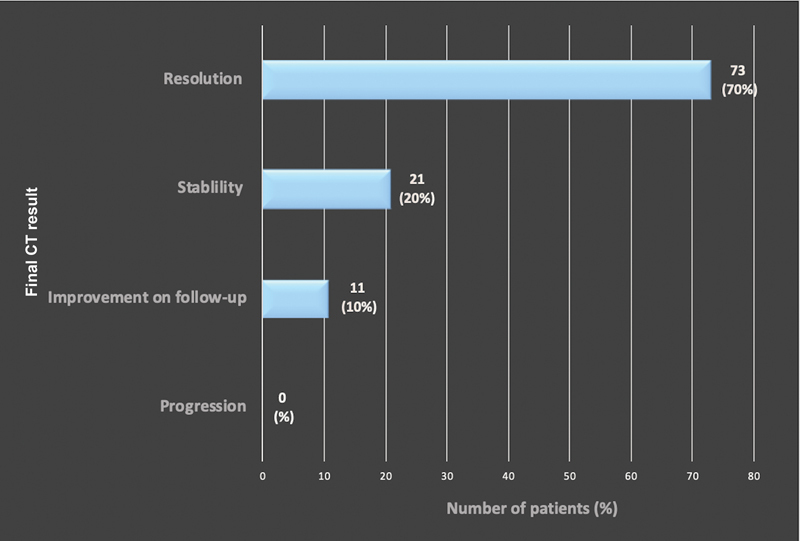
Evolution of minimal aortic injury amongst surviving trauma patients with follow-up computed tomography angiography (CTA) imaging. Seventy-three patients (70%) demonstrated resolution of minimal aortic injury (MAI) on follow-up imaging. Twenty-one patients (20%) demonstrated stability of MAI on follow-up imaging. Eleven patients (10%) demonstrated improvement on follow-up imaging.
